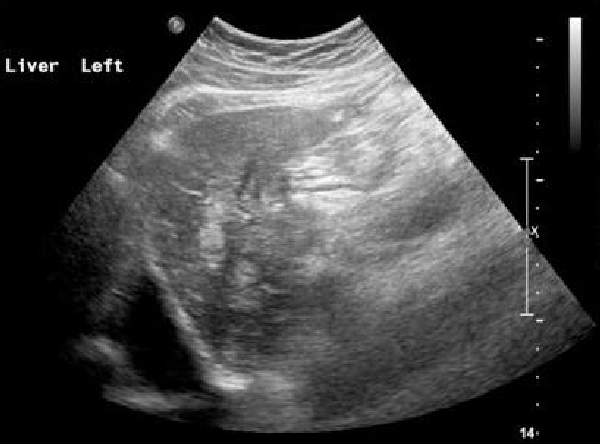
Ultrasound scan of the abdomen, demonstrating multiple echogenic foci within the liver.
